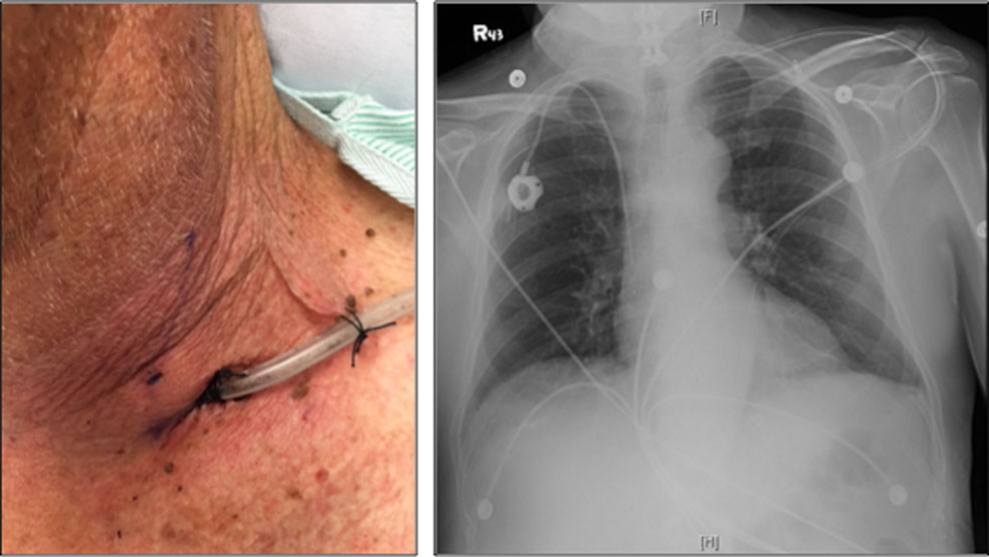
Final image of percutaneous cervical esophago-gastric tube placement along with radiographic image
